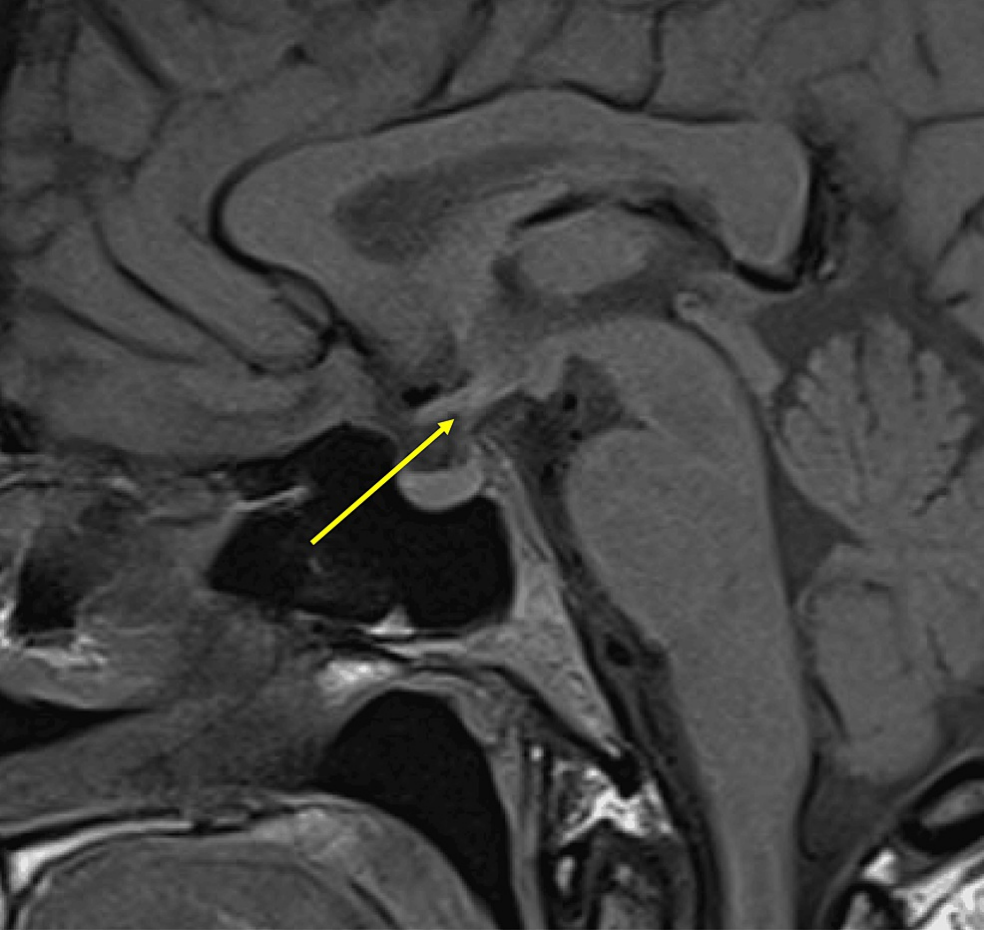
Magnetic resonance imaging section of the brain, sagittal T1 view, showing the absence of a posterior pituitary bright spot, with no evidence of posterior pituitary ectopia The yellow arrow indicates the location of the posterior pituitary, where the bright spot is absent.
